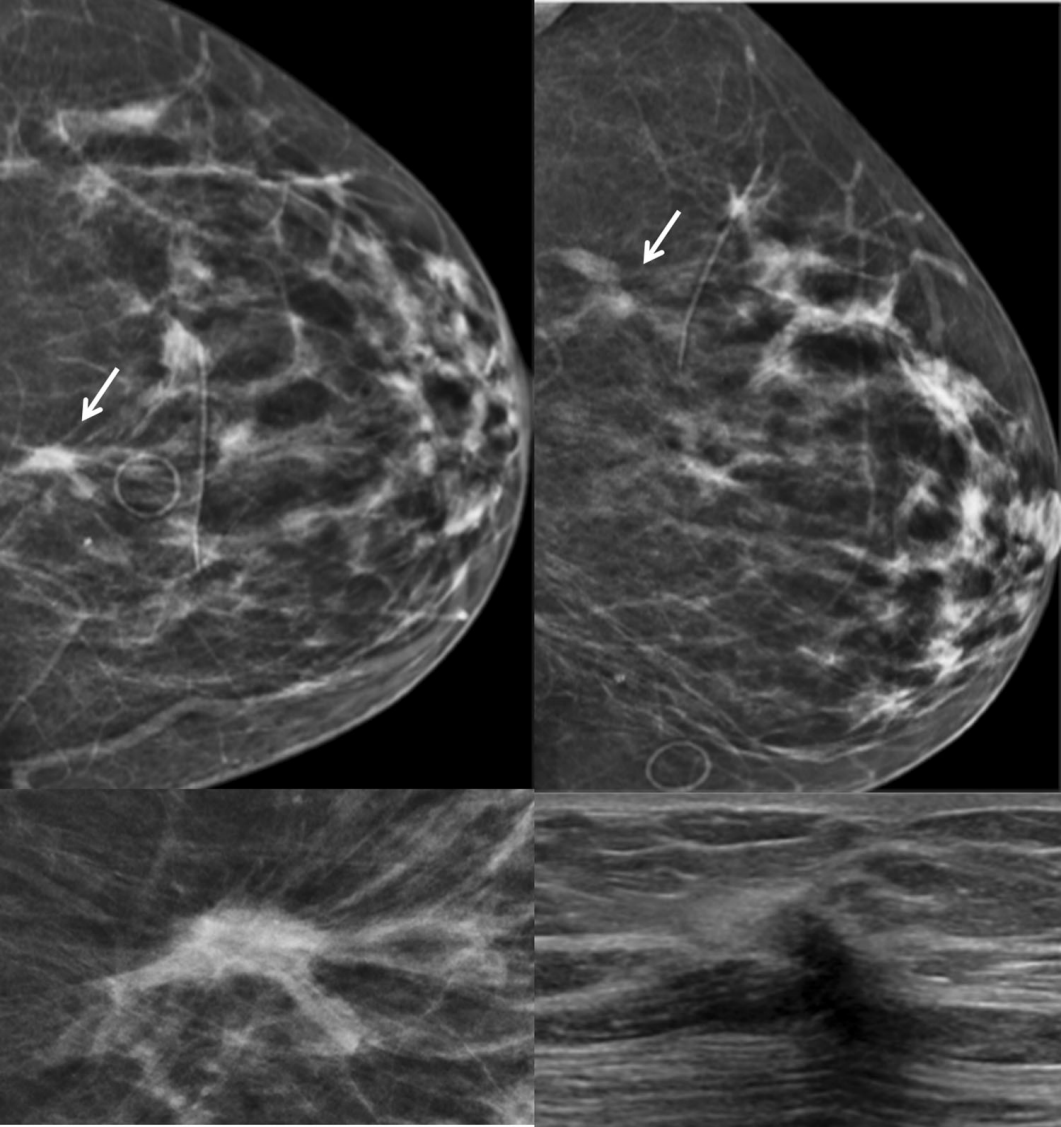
Focal asymmetry with post-surgical architectural distortion seen on mammography and ultrasound in a patient with prior breast surgery. This was initially assessed as BI-RADS 3 but subsequent follow-up mammograms demonstrate long-term stability
